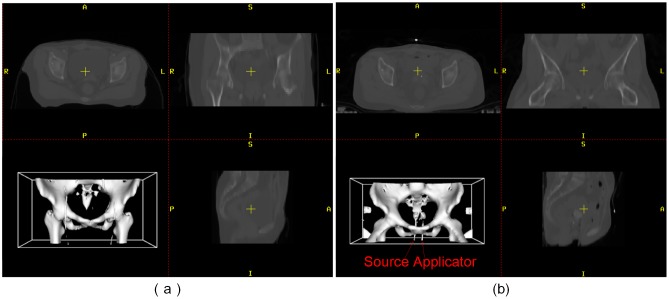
Original clinical data of one cervical cancer patient. (a) EBRT CT image. (b) HDR-BT CT image.
